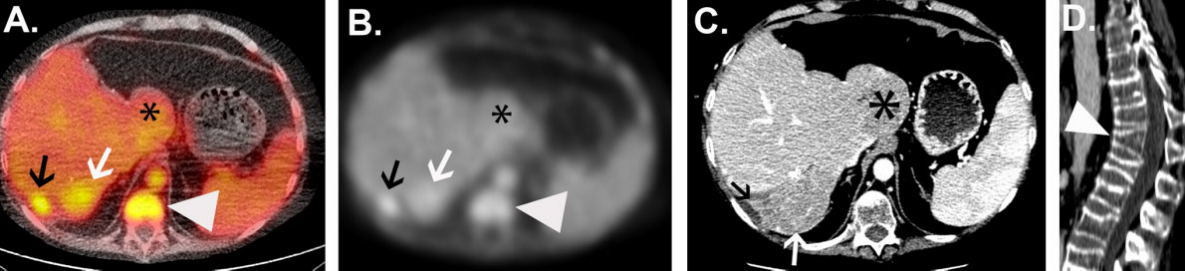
Representative images of ^68^Ga-transferrin uptake in tumor and normal structures. A, An axial PET/CT demonstrating variable uptake in 3 different hepatic masses. The most avid lesion (black arrow) corresponds to the 2.7 cm × 1.3 cm (SUV_max_ = 7.9) on the prior contrast-enhanced CT. A second lesion just medial to this (white arrow) is 5.7 cm × 5.7 cm, though had less radiotracer uptake (SUV_max_ = 6.2). A third lesion in the caudate lobe (*) measured 6.5 cm × 6.3 cm with uptake similar to uninvolved liver (SUV_max_ = 3.5). Focal uptake in the spine (white arrow head) corresponds to the vertebral body compression deformity seen on sagittal CT. B, The corresponding CT collected during the PET/CT acquisition. C, The contrast-enhanced CT collected 1 month prior to the PET/CT. D, A sagittal view of the CT showing the vertebral body compression. CT indicates computed tomography; PET, positron emission tomography; SUV_max_, maximum standardized uptake value.
